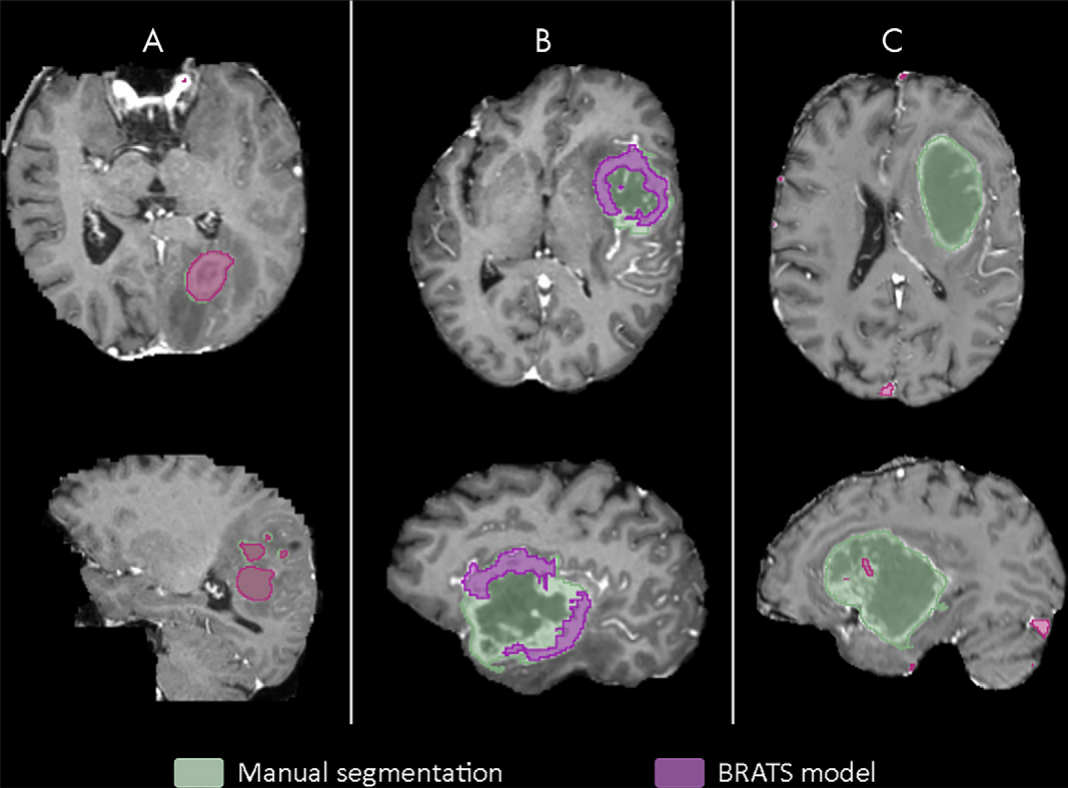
Segmentations generated by the Multimodal Brain Tumor Image Segmentation (BraTS) model overlaid on the manual segmentations for, *A*, BraTS test patient (Dice score, 0.85), *B*, clinical patient with complete secondary imaging (Dice score, 0.43), and, *C*, patient with missing T2-weighted and T2-weighted fluid-attenuated inversion recovery images (Dice score, 0.001).
